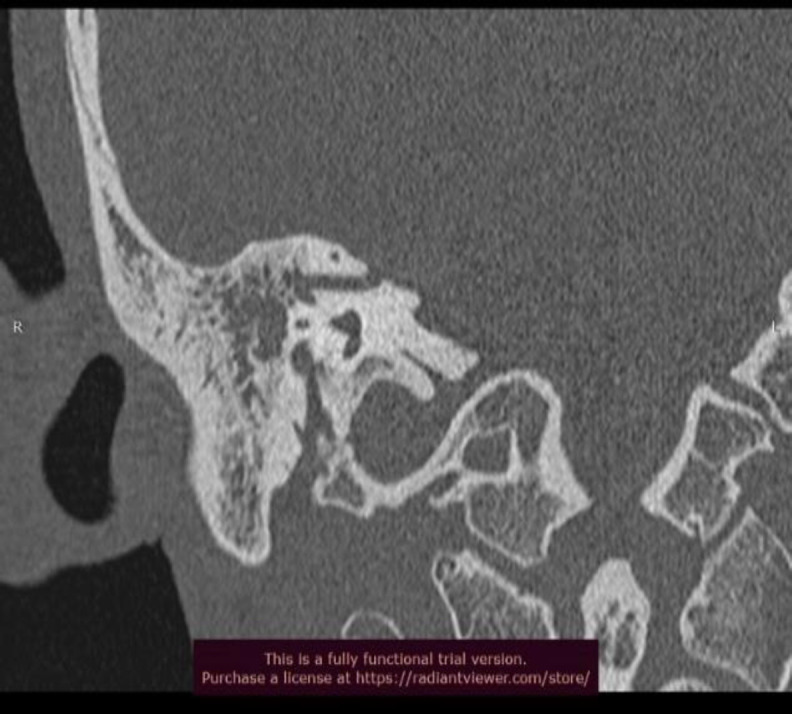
Coronal section of HRCT Temporal Bone showing dilated and anomalous petromastoid (subarcuate) canal
